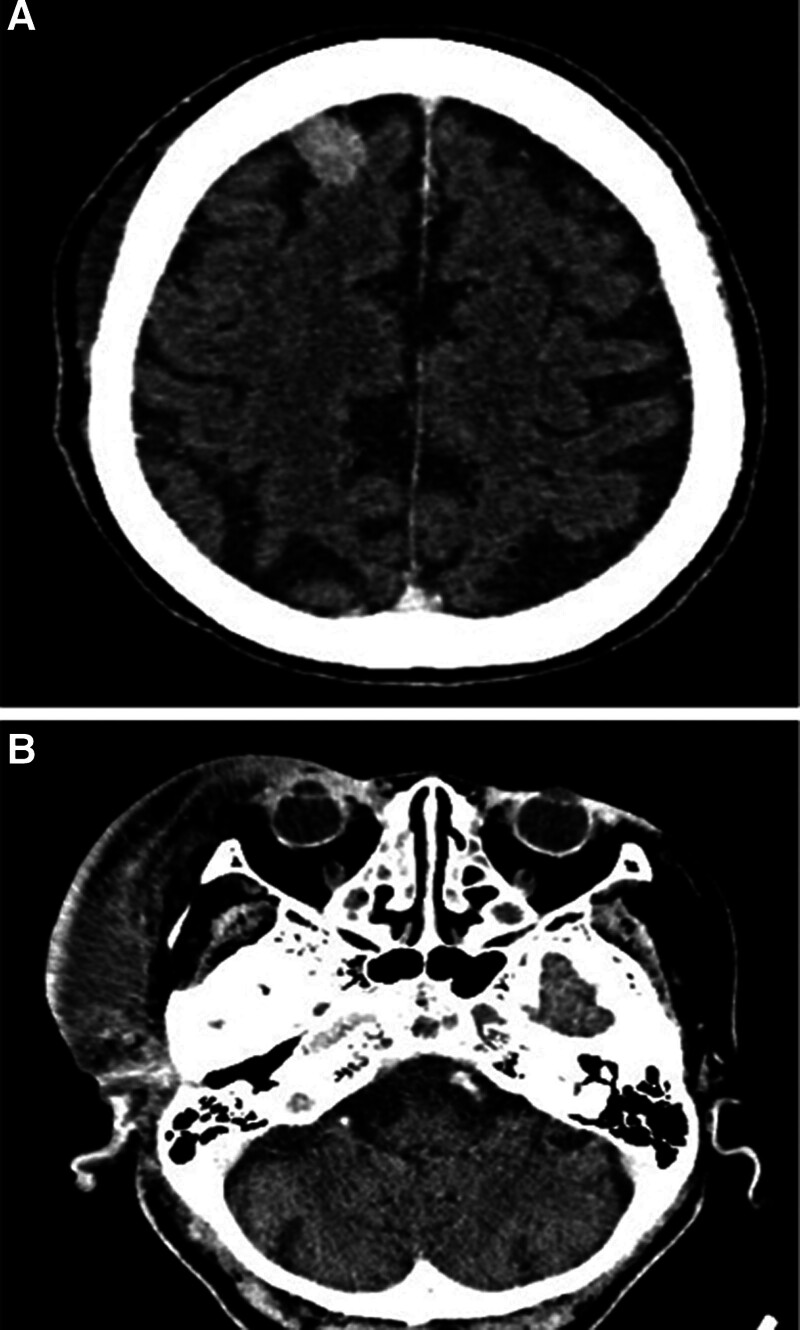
(A, B) CECT showed large right hemifacial and right scalp cellulitis with focal abscess at right temporal area. CECT = contrast-enhanced computed tomography.
